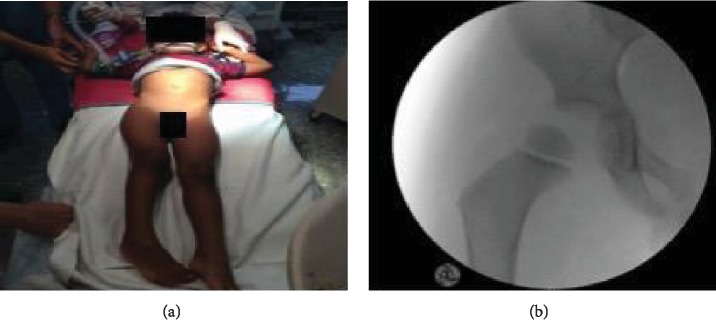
(a, b) Clinical findings before reposition and postinterventional image intensifier a.p. radiograph showing the incomplete reduction with persisting subluxation.
